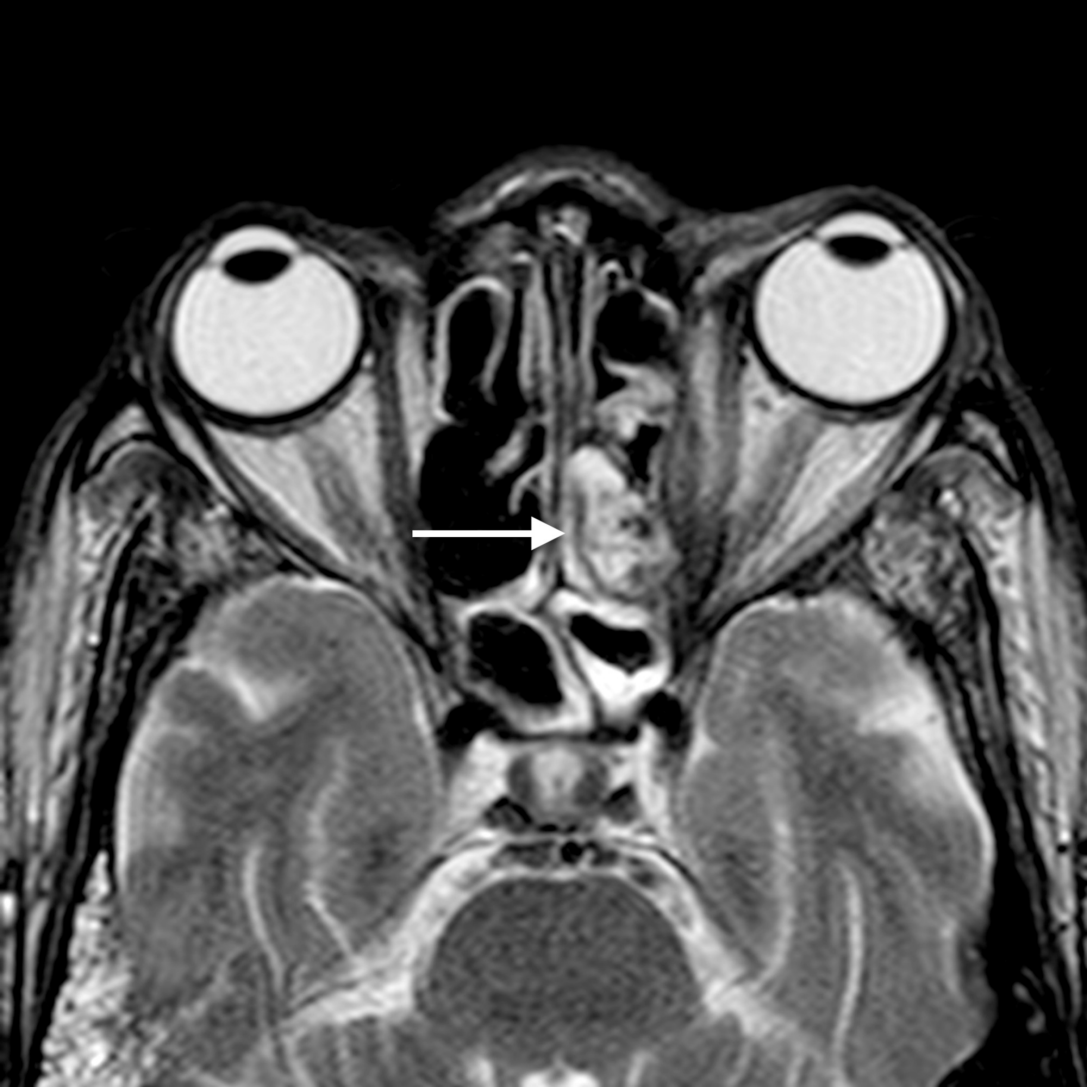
Orbital cellulitis: axial T2WI shows abnormal soft tissue in the left posterior ethmoid air cells (arrow) appearing hyperintense TW2I - T2-weighted image
